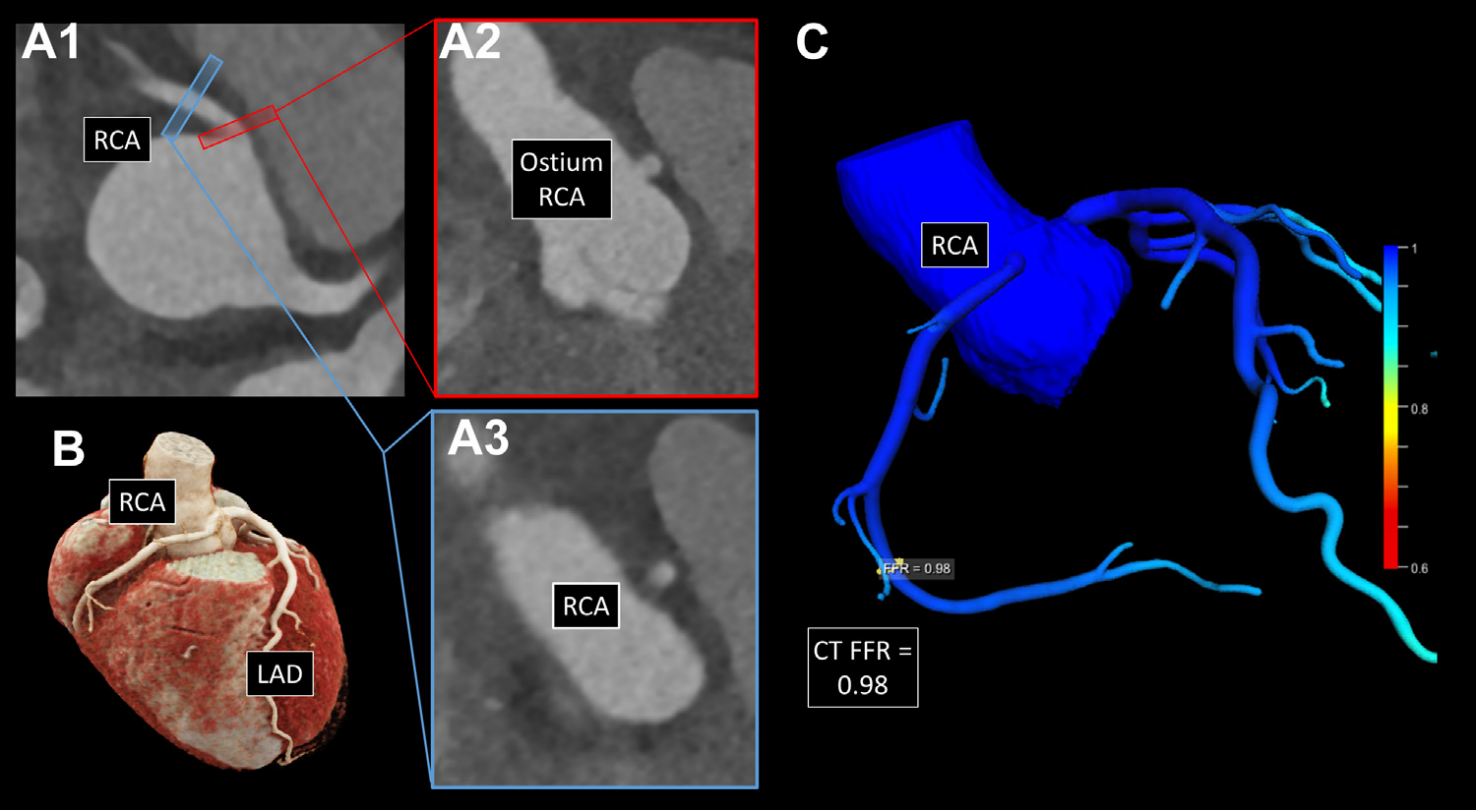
Postoperative Diagnostic Evaluation (A1) Coronary computed tomography angiography (CCTA) of the right anomalous coronary artery originating from the opposite sinus of Valsalva (R-ACAOS) after surgical unroofing. (A2) Depiction of the R-ACAOS origin demonstrating successful correction of the slitlike ostium. (A3) Unchanged distal reference diameter of the right coronary artery (RCA). (B) Three-dimensional reconstruction of the CCTA without visible external alterations because of the applied surgical procedure, ie, surgical unroofing of the intermural course. (C) Postoperative CCTA-derived fractional flow reserve (CT FFR) demonstrating the hemodynamic success of the surgical procedure.
